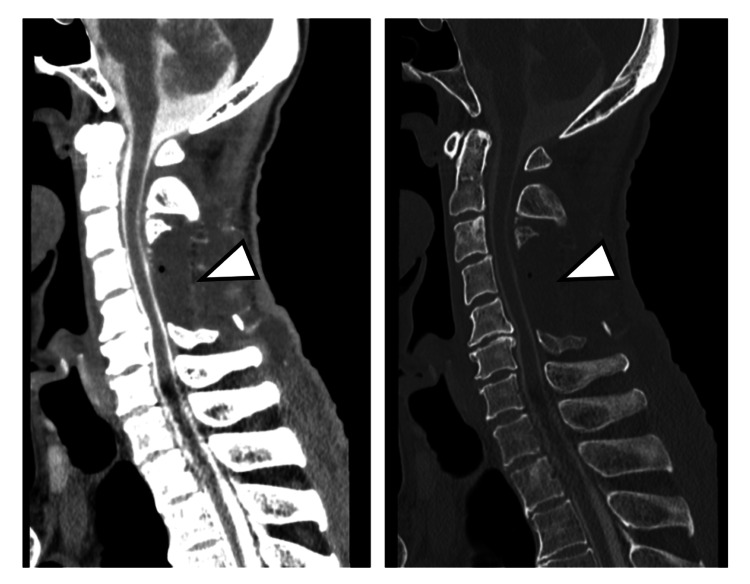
Cervical myelography CT (sagittal image) obtained 10 days after operation No apparent cervical epidural contrast leakage was observed (arrowhead) CT: computed tomography
